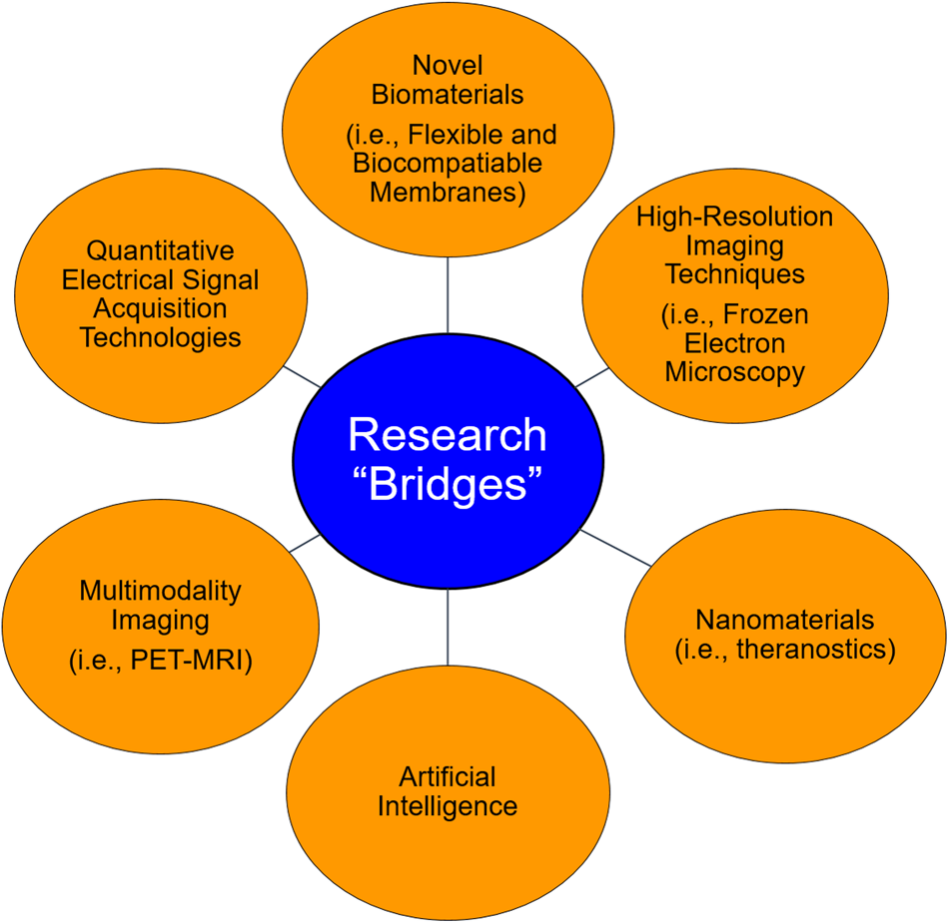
Building research “bridges” to overcome the reverse inference fallacy and advance the study of MRI in major depressive disorder (MDD) may be accomplished using several strategies, including novel biomaterials, high-resolution and multimodality imaging techniques, artificial intelligence, novel nanomaterials, and quantitative electrical signal acquisition technologies.
